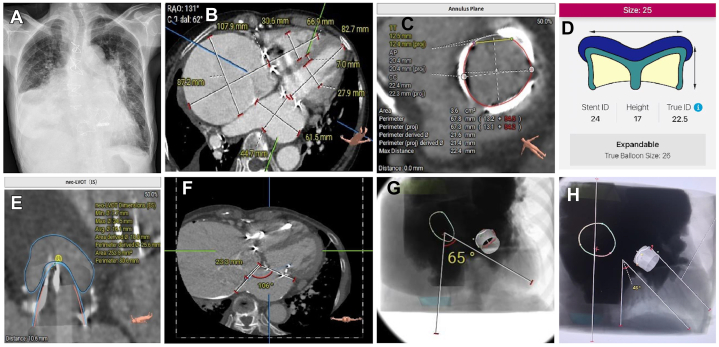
Procedural Challenge (A) Significantly increased cardiothoracic ratio. (B) Preprocedural computed tomography angiography showing the massive atrium. (C and D) True internal diameter of the bioprosthesis measured by computed tomography angiography and the baseline data. (E) Neo–left ventricular outflow tract area. (F) Septal-annular angle. (G) Angle between the inferior vena cava–atrial septum line and the mitral valve–left ventricular apex axis. (H) Angle between the inferior vena cava–superior vena cava axis and the mitral annulus–left ventricular apex line.
